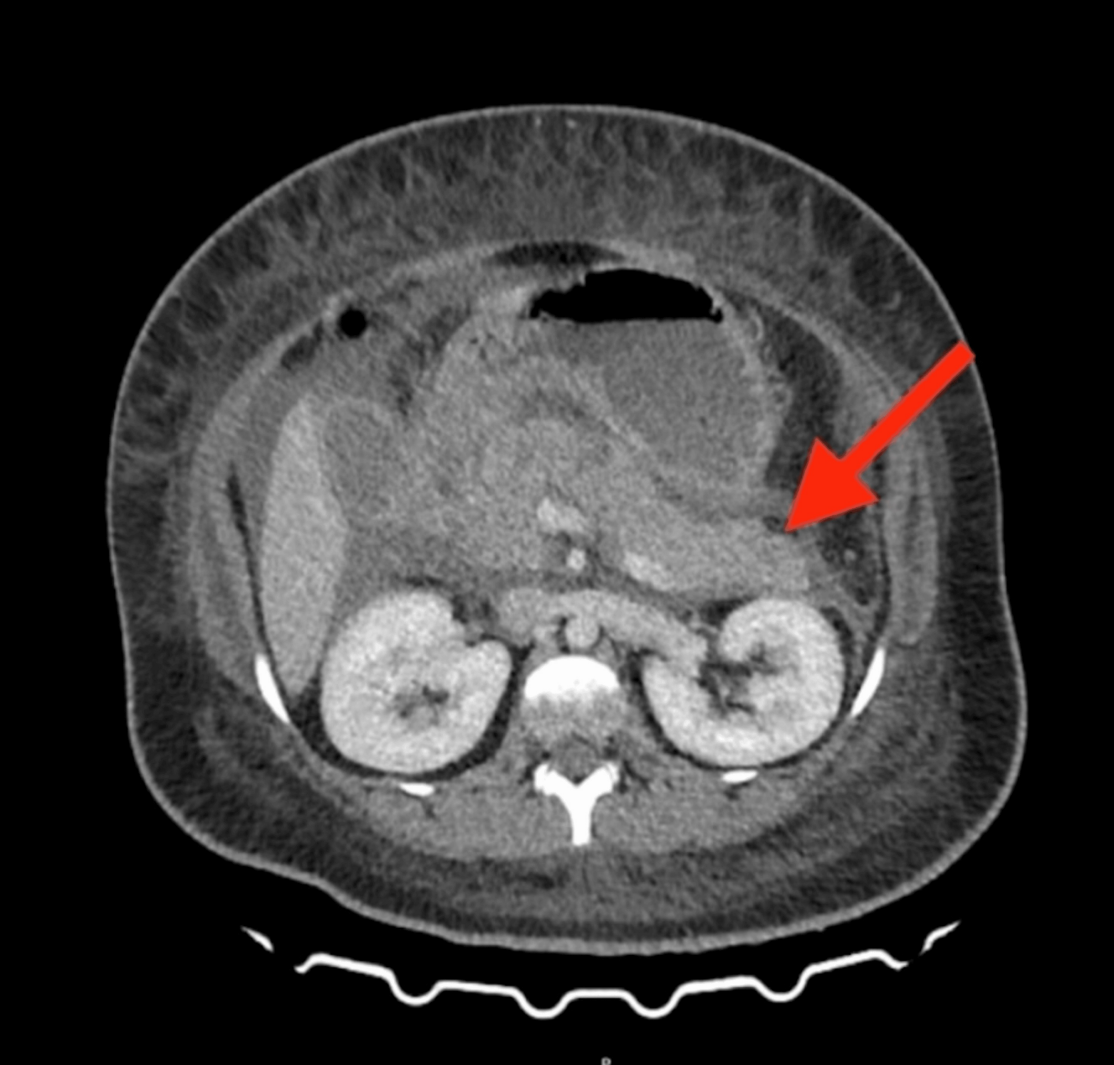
CT scan of abdomen and pelvis with contrast showing bulky pancreas with peripancreatic fat stranding and non-enhancing necrotic areas seen. Peripancreatic fluid noted extending to anterior renal fascia, para-colic gutters and forming a collection in pelvis.
